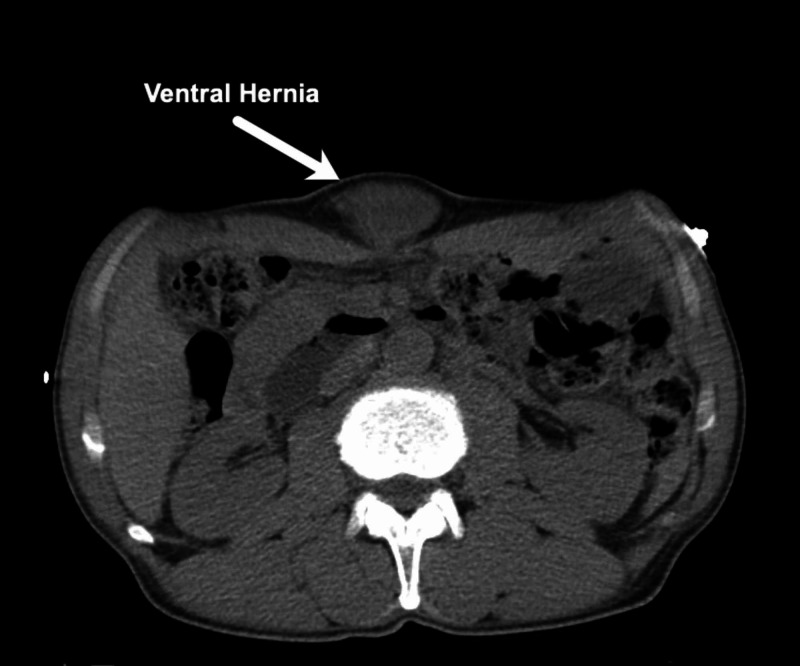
Case 2: Coronal CT scan.
